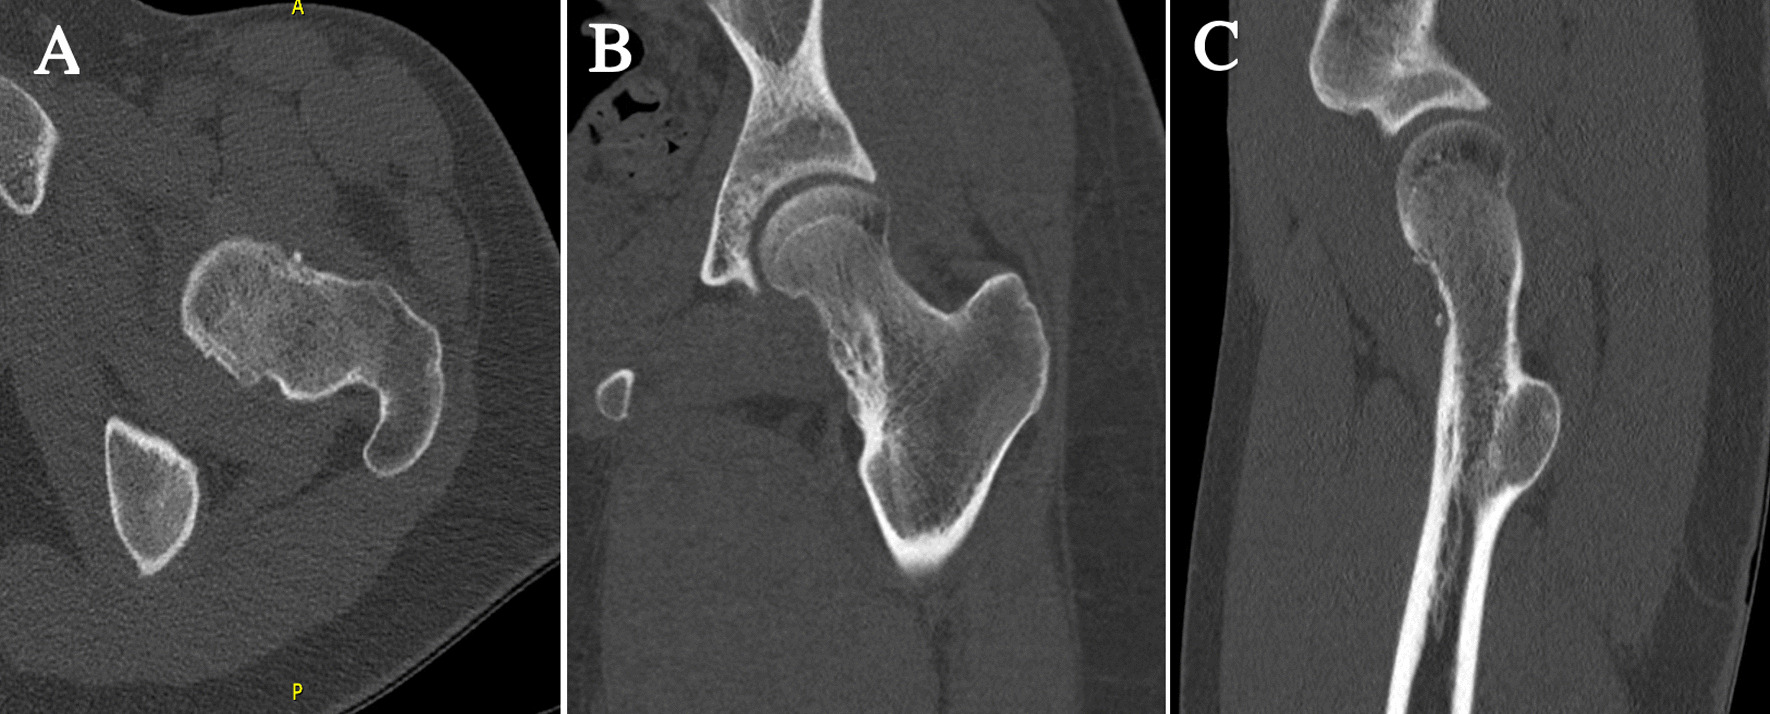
A Axial, B coronal, and C sagittal CT scans of the left hip at the 6-month follow-up visit showing complete excision with no recurrence
